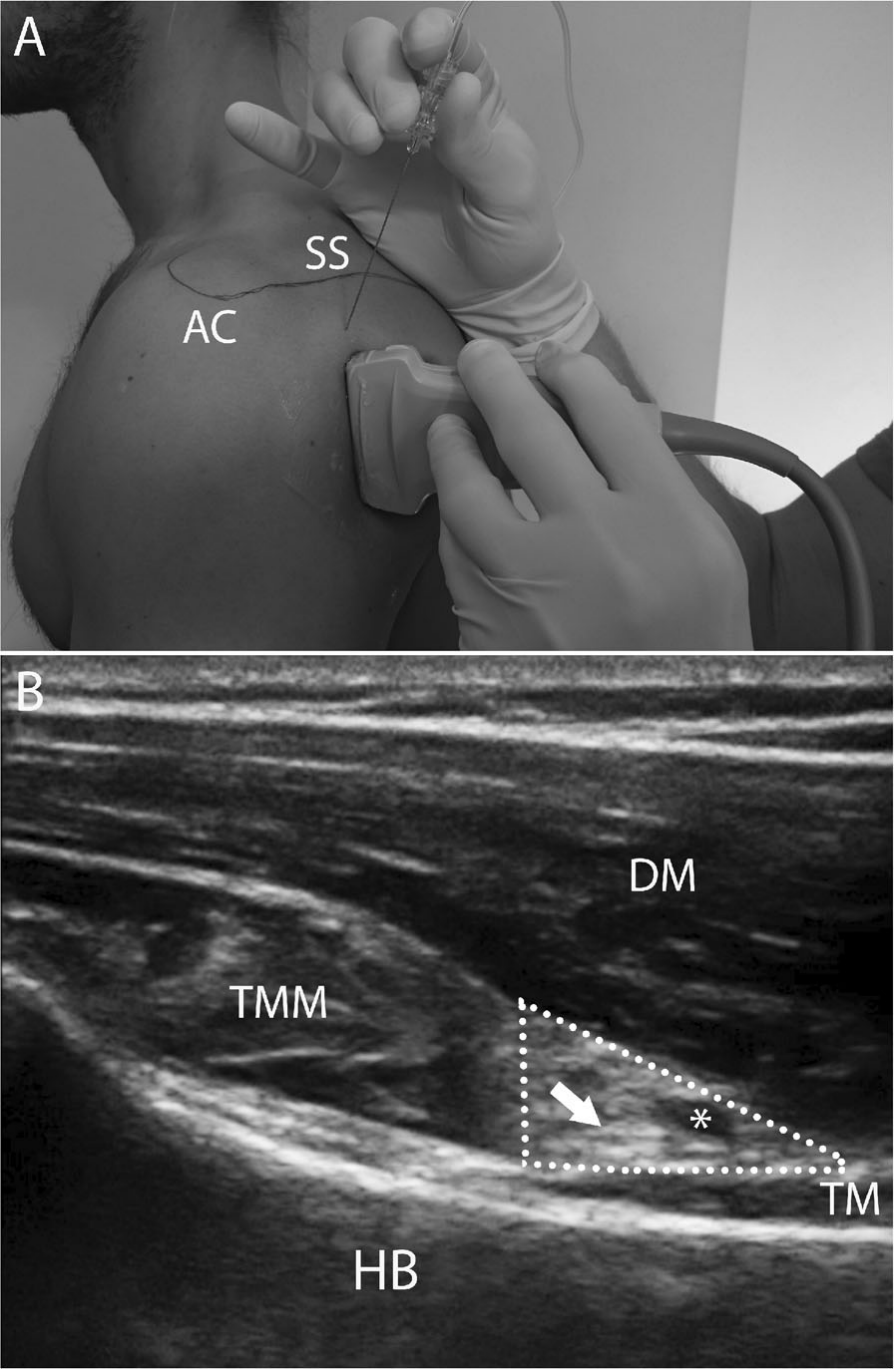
a Surface anatomy and transducer position of selective axillary nerve block. The acromion (AC) and the scapular spine (SS) are marked. b Ultrasonographic image of the shoulder region with the transducer positioned as in Fig. 1. a Important landmarks are the deltoid muscle (DM), the humeral bone (HB), the teres minor muscle (TMM) in transverse section and the triceps muscle (TM) in longitudinal section. The axillary nerve (arrow) is cranial to the posterior circumflex humeral artery (*) in the neurovascular space (dotted triangle)
